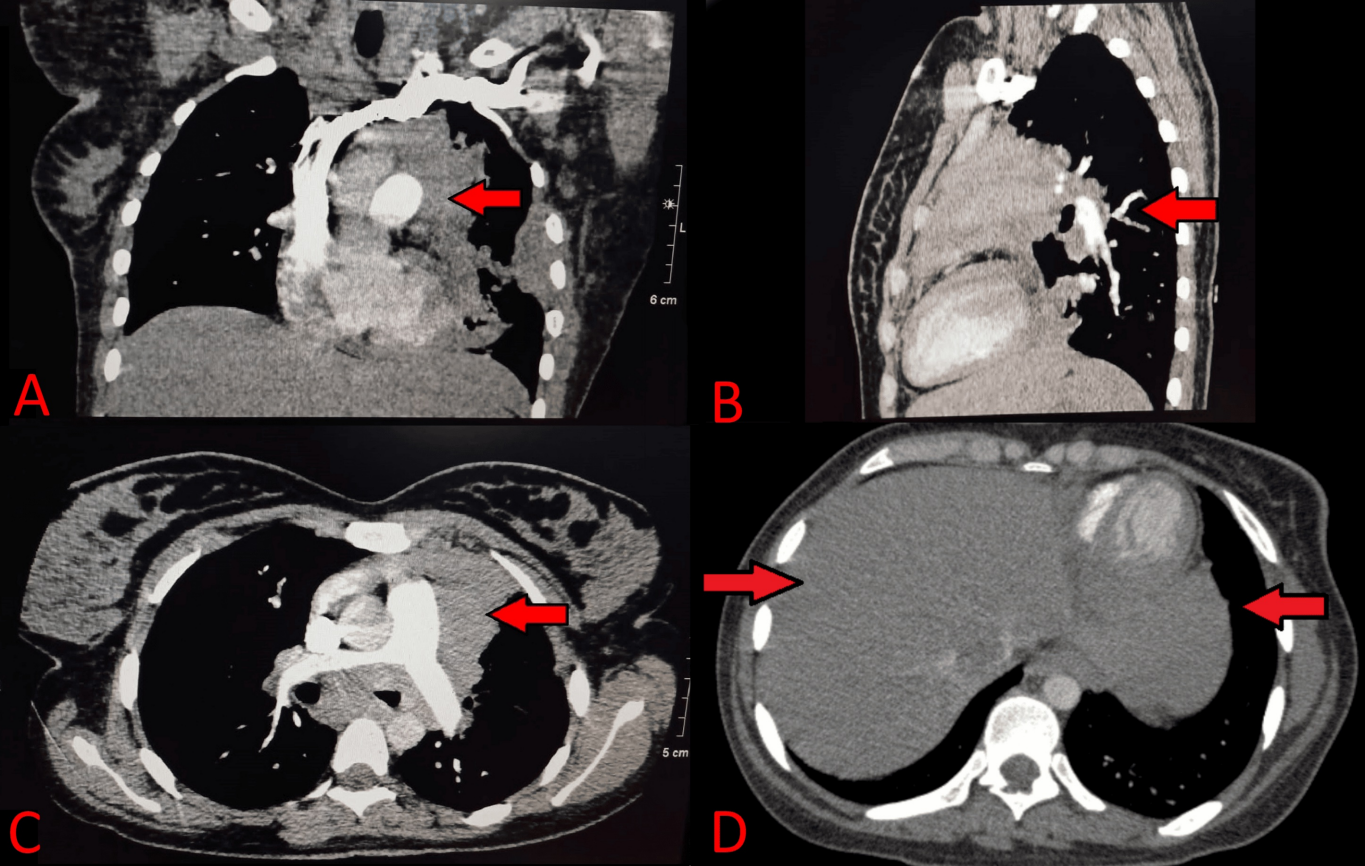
Chest CT scan (A) Coronal view, (B) sagittal view, and (C) axial view of the expanding pulmonary and mediastinal mass. (D) Right lung involvement along with the left.
